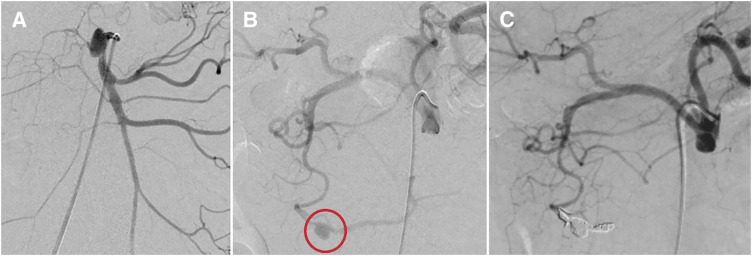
Angiographic findings and endovascular treatment. (A) SMA angiography: Dissection is noted at the origin, with the dissection cavity appearing aneurysmal, consistent with computed tomography findings. (B) CA angiography: An aneurysm (circle) is visualized in the anterior superior pancreaticoduodenal artery. No contrast extravasation or active bleeding is observed. (C) Endovascular treatment: A 5-Fr long sheath was inserted, and a Shepherd’s hook catheter was placed in the CA. Using a microcatheter, the aneurysm was isolated distally with metallic coils. CA: celiac artery; SMA: superior mesenteric artery
